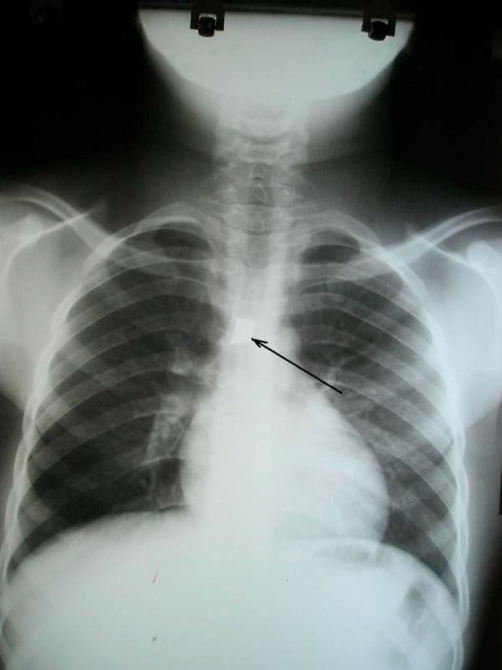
Anterior-posterior chest X-ray showing the radio-opaque foreign body in right main bronchus (Arrow).
